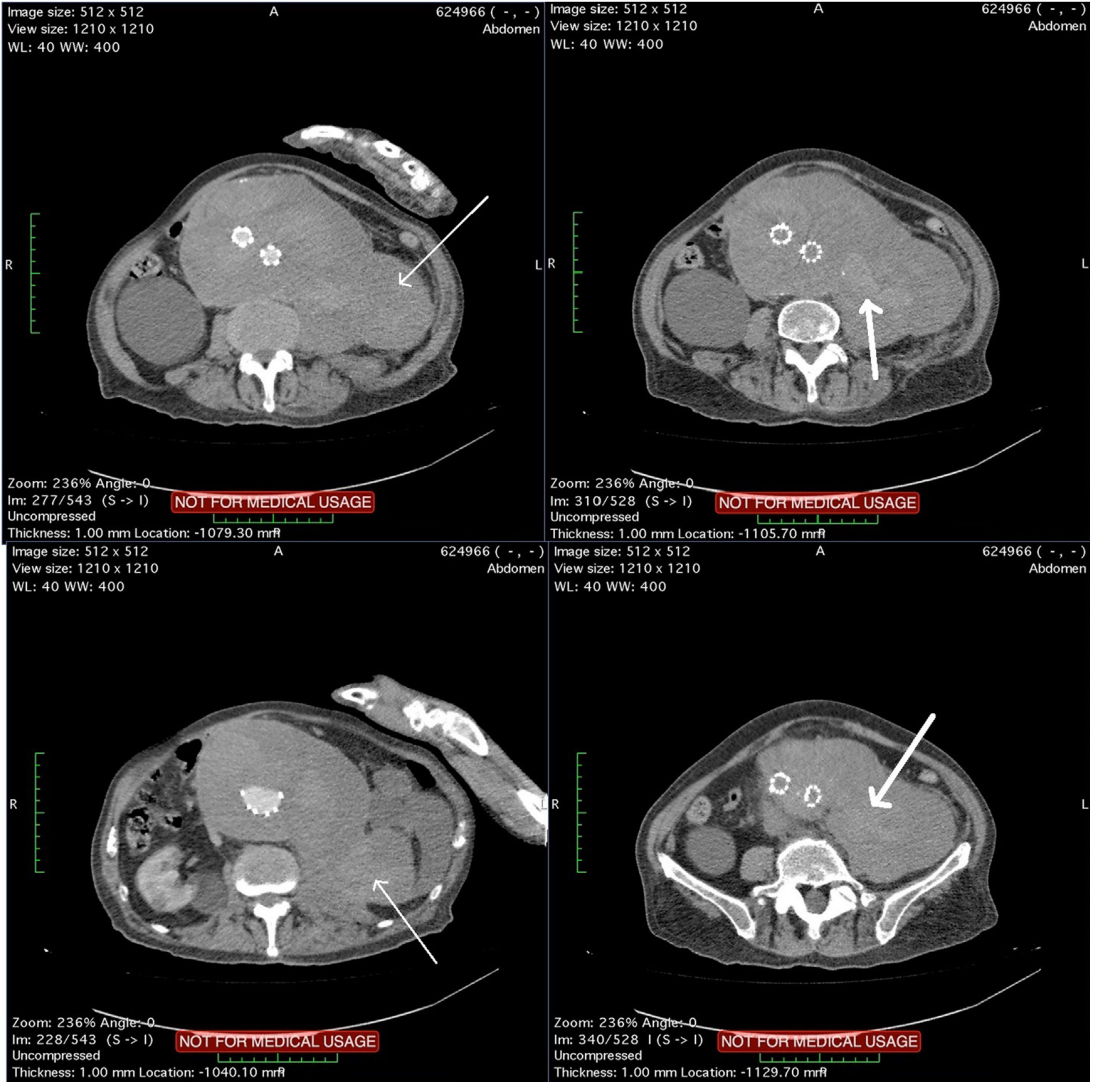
Computed tomography angiography images showing a rupture of the aneurysm sac that causes a large retroperitoneal hematoma (thin arrows). Blood pools are observed within the hematoma (thick arrows) although no typical endoleak can be identified.
